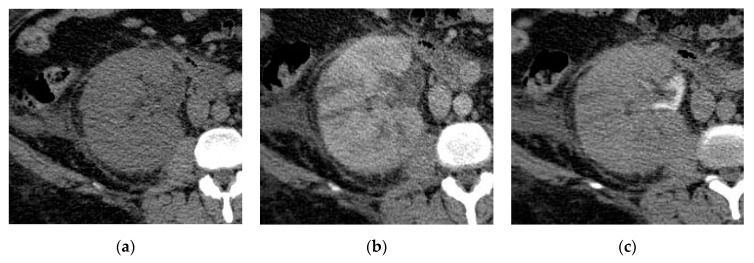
Acute right pyelonephritis. (a) Axial non-contrast computed tomography (CT) image shows enlarged right kidney with an irregular outline, thickened perinephric fascia, and distorted perinephric fat. Axial (b) early and (c) delayed post-contrast CT images show linear streaks extending from the papillae to the cortex with an impaired enhancement of the parenchyma.
